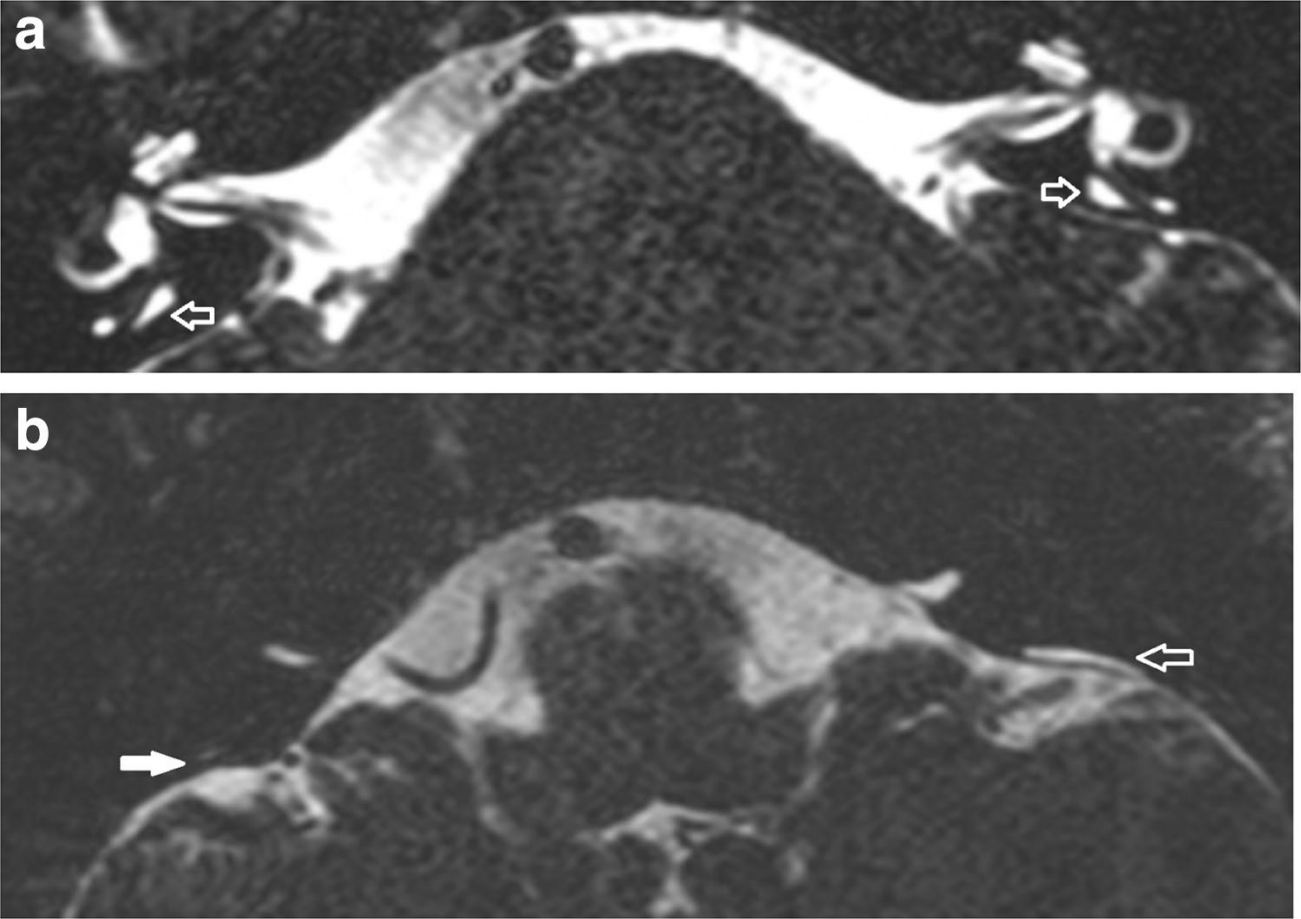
Intra-osseous measurements diagnose LESA on MRI but extra-osseous sac not enlarged. T2 DRIVE axial images show widened midpoint measurements bilaterally (white open arrows in a). There is an enlarged extra-osseous sac on the left (white open arrow in b) but not on the right (white filled arrow in b)
